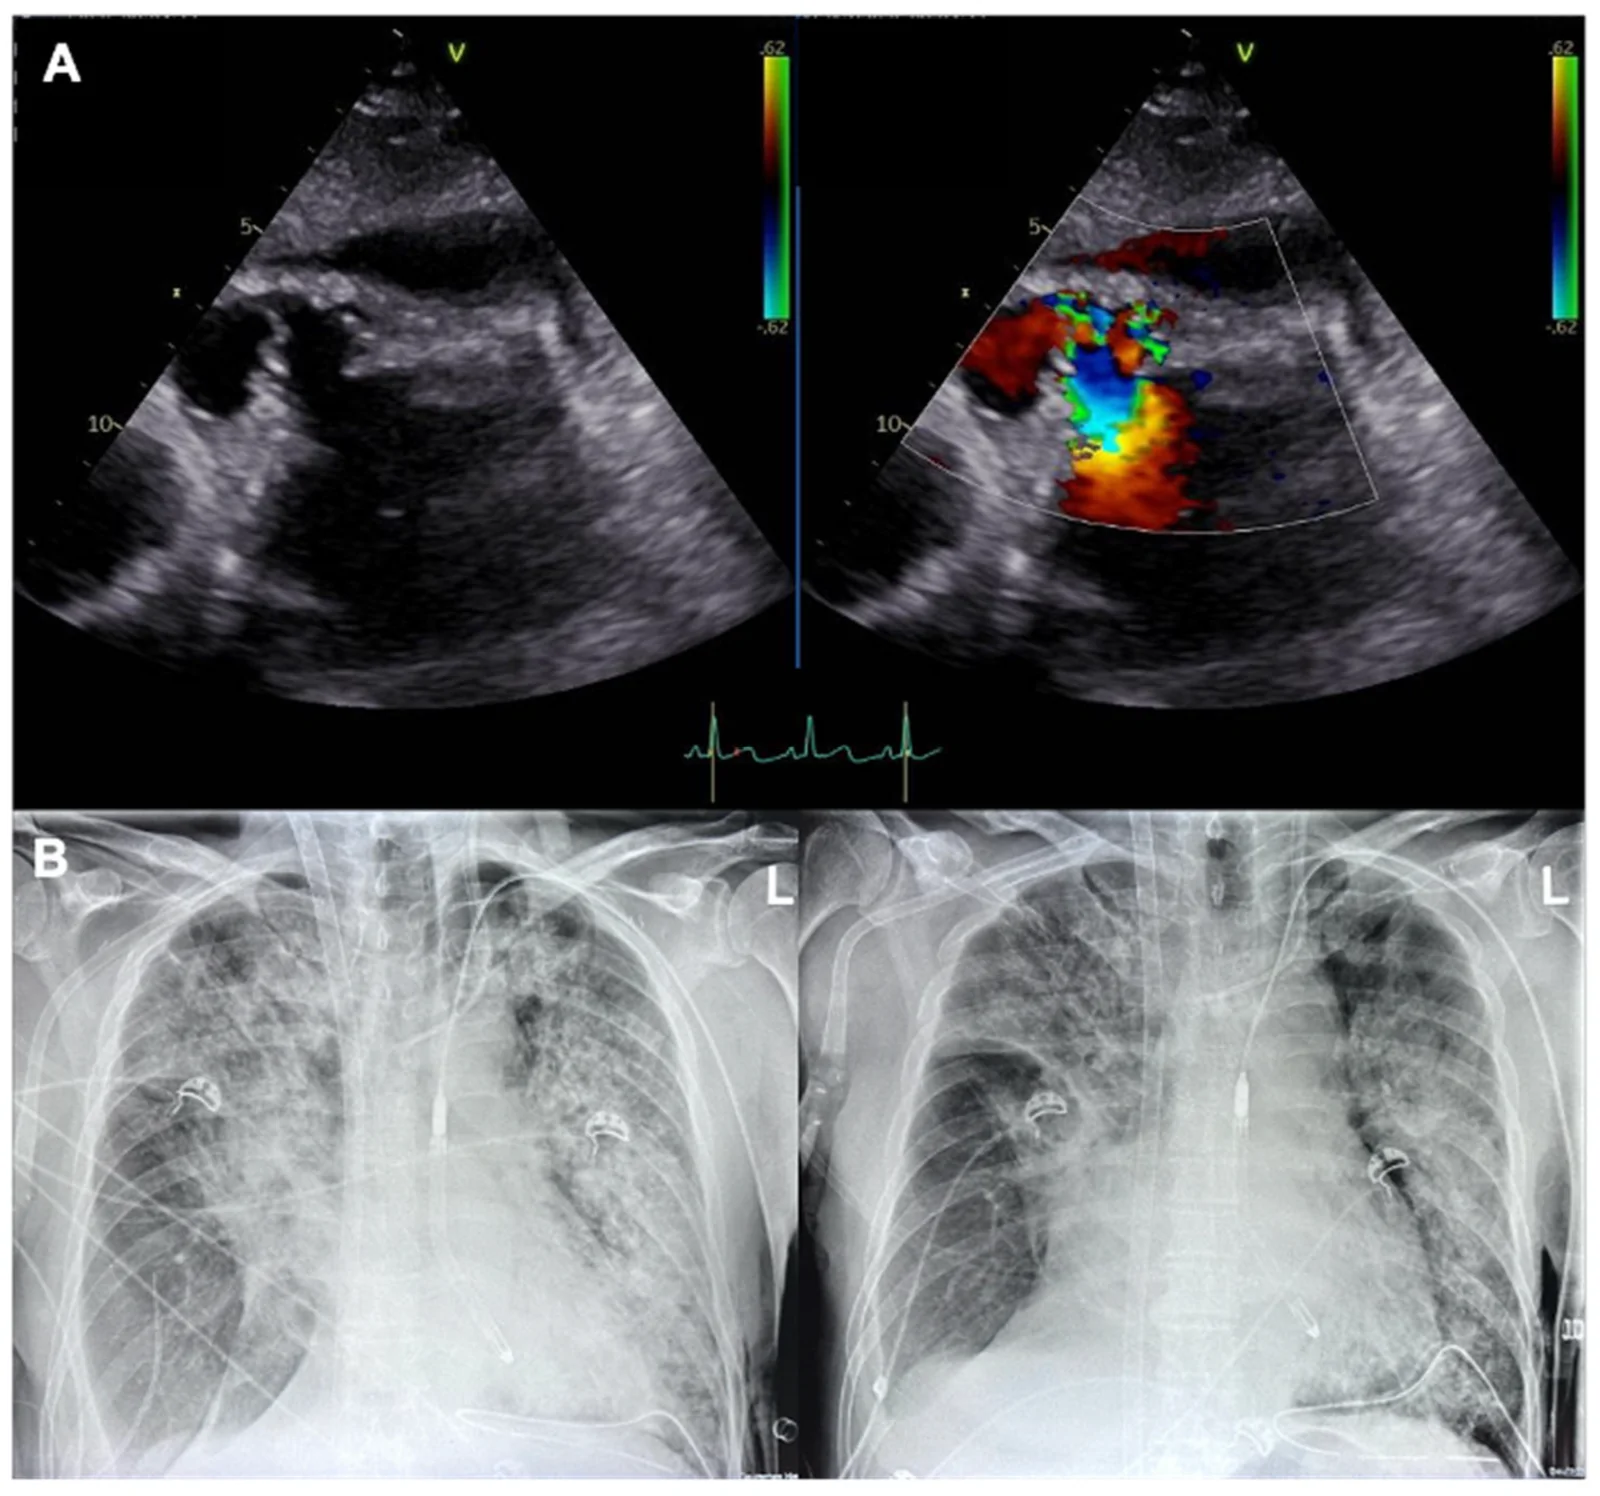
(A) Subxiphoid transthoracic echocardiographic view demonstrating a post-infarction ventricular septal defect with left-to-right shunting on color Doppler imaging. (B) Hemodynamic stabilization with a combination of Impella 5.5 with VA-ECLS (ECMELLA 2.1: single artery access with jugular venous cannulation [13]) resulted in improvement in pulmonary edema, as demonstrated on follow-up chest radiography.
